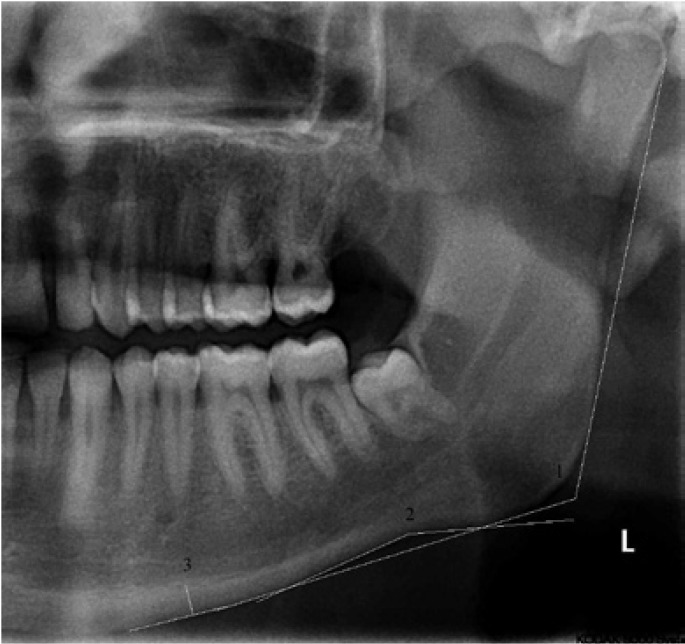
Cropped panoramic radiograph showing the gonial angle (1), antegonial angle (2) and mandibular cortical width (3).
